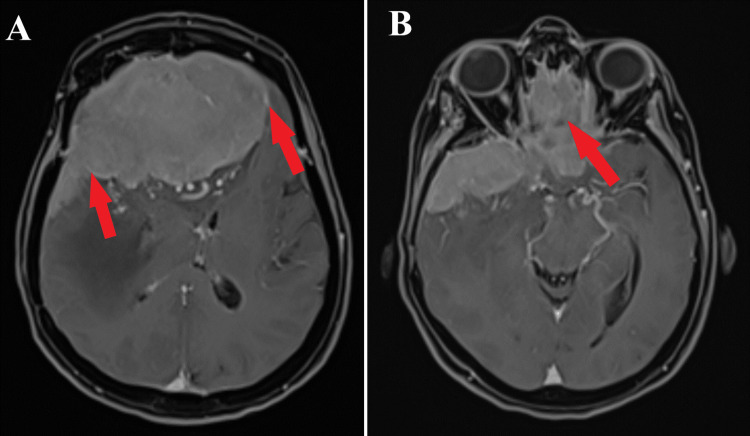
Preoperative MRI T1 post contrast sequence, axial section. A: Grasping post gadolinium administration is seen (red arrows).
